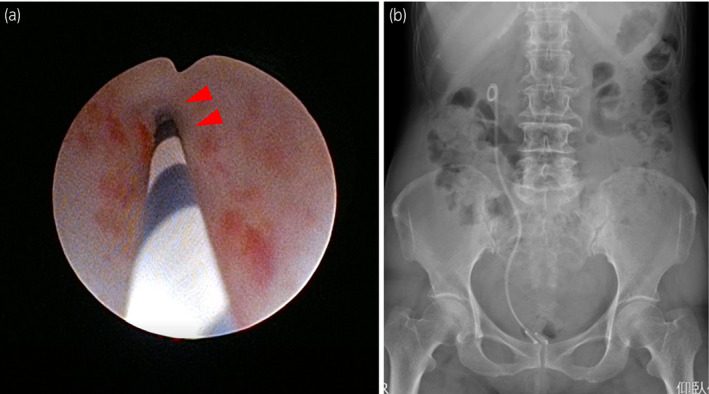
Ureteral stricture seen by URS (a; red arrowhead). Dual stent placement, as visualized by plain abdominal radiograph (b).
